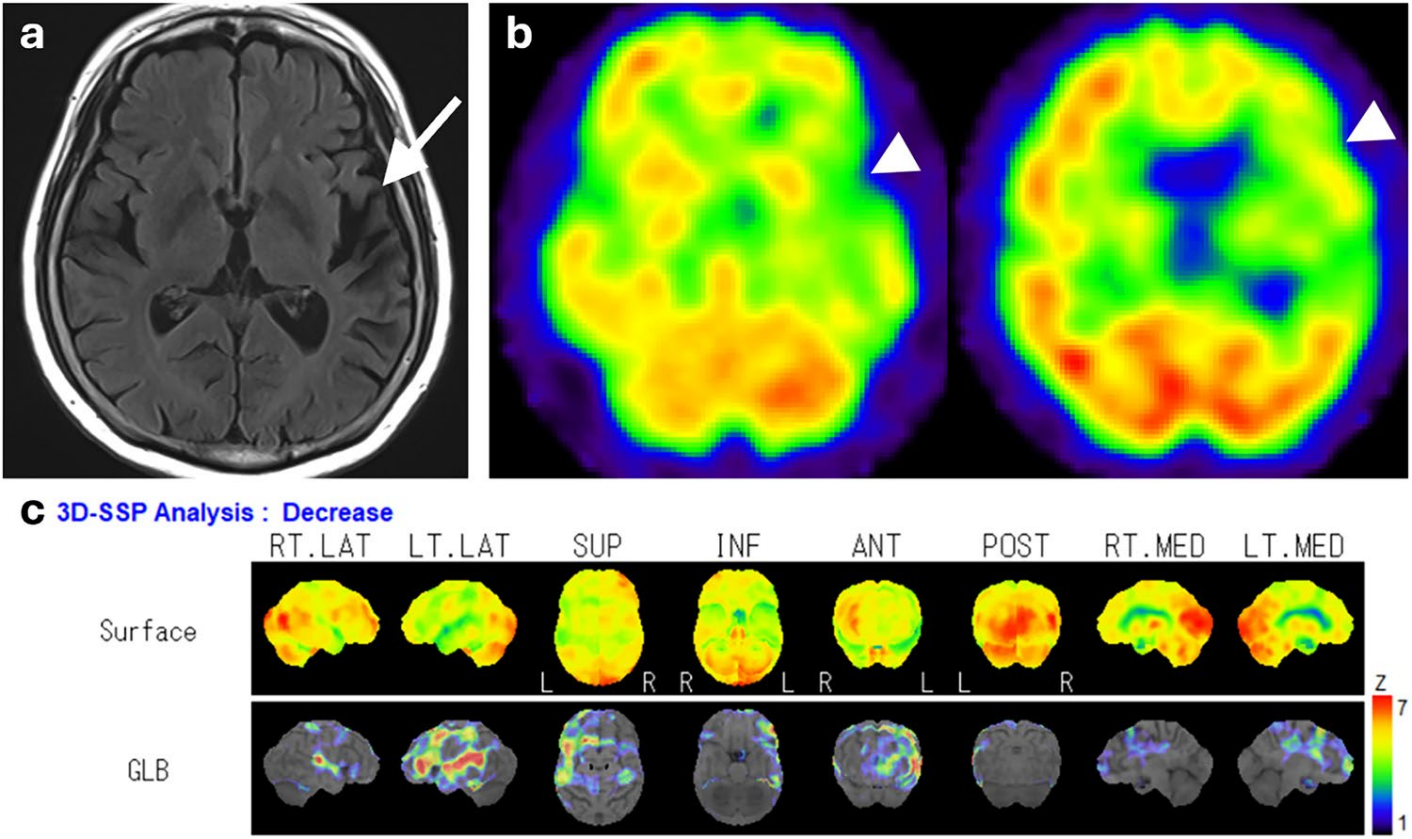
A 66-year-old female with PNFA. a Axial FLAIR images showed a subtle enlargement of the left Sylvian fissure (arrow). b IMP brain perfusion images and c 3D-SSP analysis demonstrated hypoperfusion in the posterior left frontal lobe extending to regions around the left Sylvian fissure, consistent with PNFA (arrowheads)
